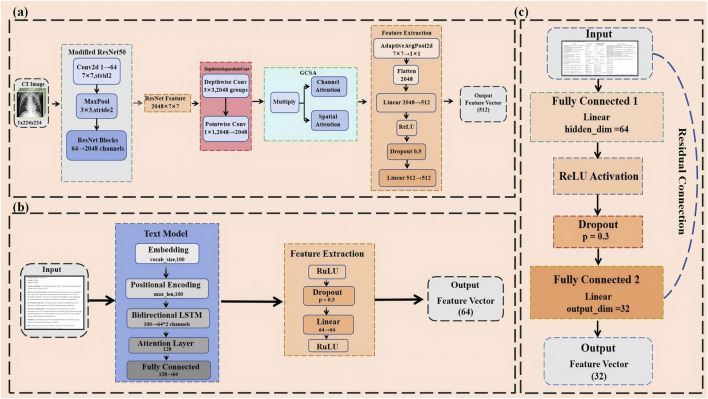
(A) Modified ResNet50-Based CNN for hierarchical CT image feature extraction with GCSA and depthwise separable convolutions. (B) BiLSTM-attention based text model for multimodal clinical and radiological data integration. (C) Mlp with residual connections for numerical data feature processing.
